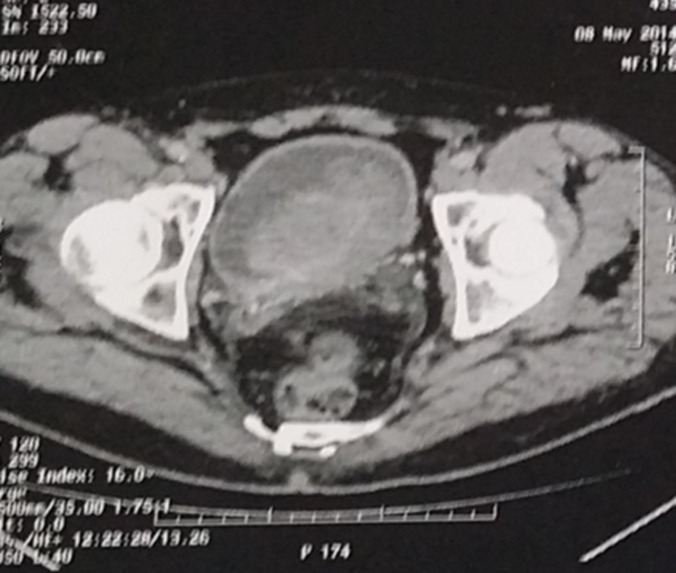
CT scan showing a huge bladder tumor invading the peri-bladder fat classified T3N0M0
